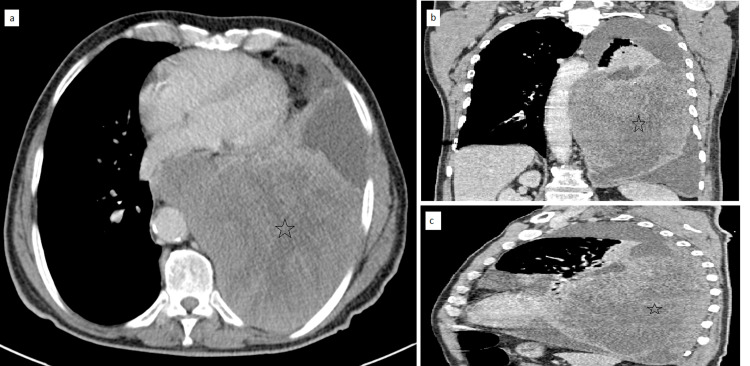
Axial (a), coronal (b), and sagittal computed tomography (c) scans showed a voluminous left lower lobe tumor process (black star) with lobulated margins, heterogeneous, and enhanced after contrast, measuring approximately 135 x 116 x 25 mm.
